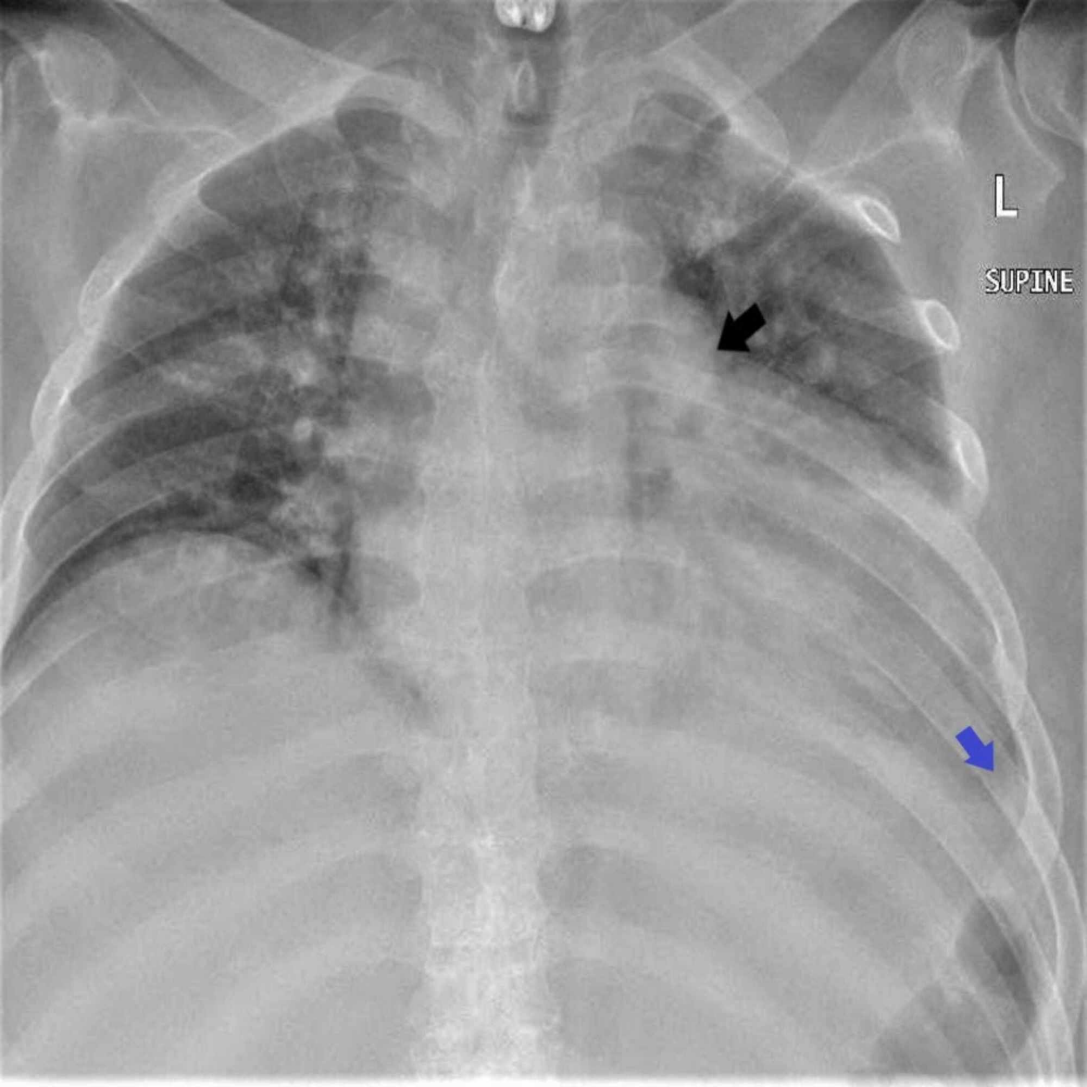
X-ray revealing a large pericardial effusion with features of tamponade The black arrow points towards the increased cardiac silhouette suggestive of pericardial effusion; the blue arrow shows a left blunted costophrenic angle due to pleural effusion.
